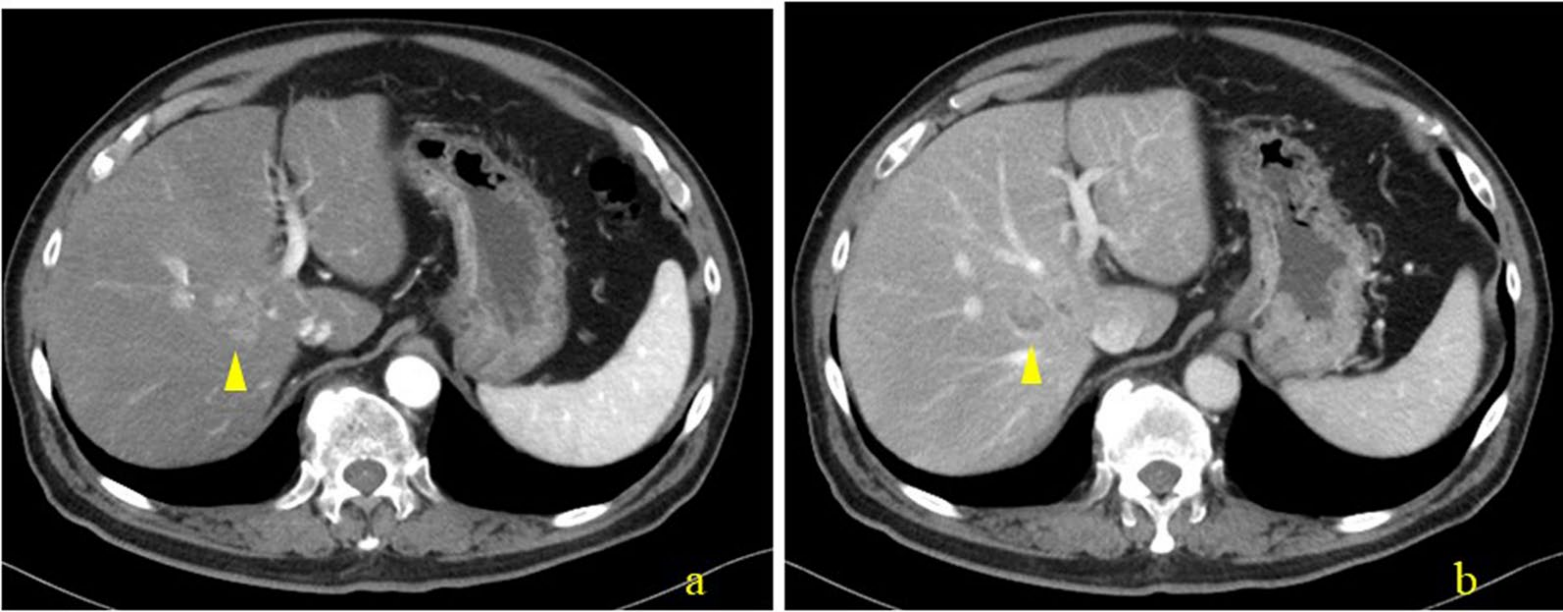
Abdominal contrast-enhanced computed tomography. a Tumor showed a heterogeneous contrast effect in the arterial phase, located at the hepatic hilum, slightly cephalad, and dorsal to the right hepatic duct (arrowhead). b Tumor showed prolonged enhancement at the late phase, accompanied partly by a low attenuated component mimicking cyst-like structures
